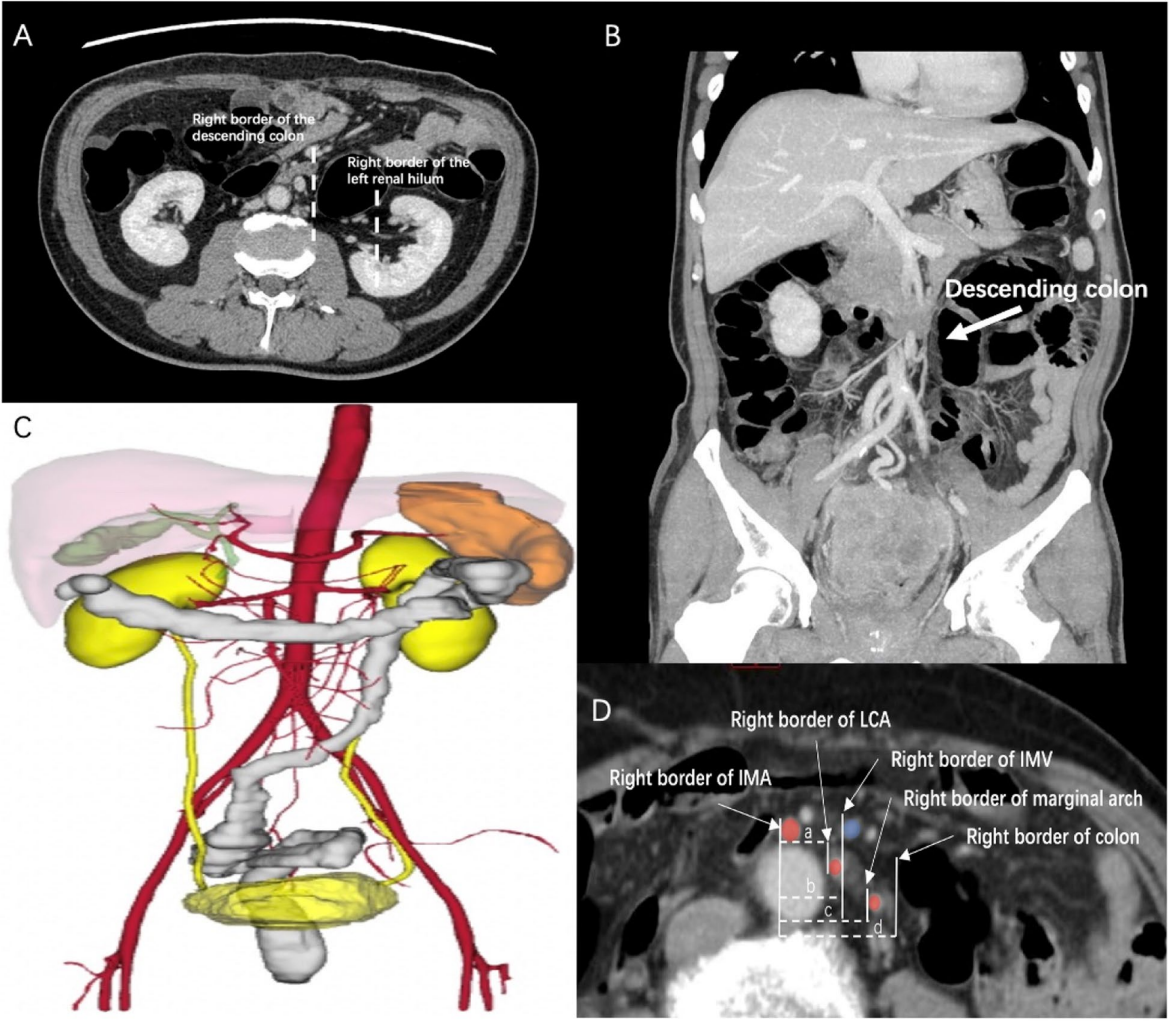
The characteristics of PDM in the CT scan. A CT Axis, PDM was defined as “the right margin of the descending colon located in the left renal hilum.” B coronal CT scan: the descending colon near the midline of the abdomen. C 3D reconstruction: the sigmoid colon shifted to the right side of the abdomen. D Measurement of various lengths at the IMA root level: a. length between the IMA and LCA. b. length between the IMA and IMV c. length between the IMA and marginal arch. d. length between the IMA and the colon
